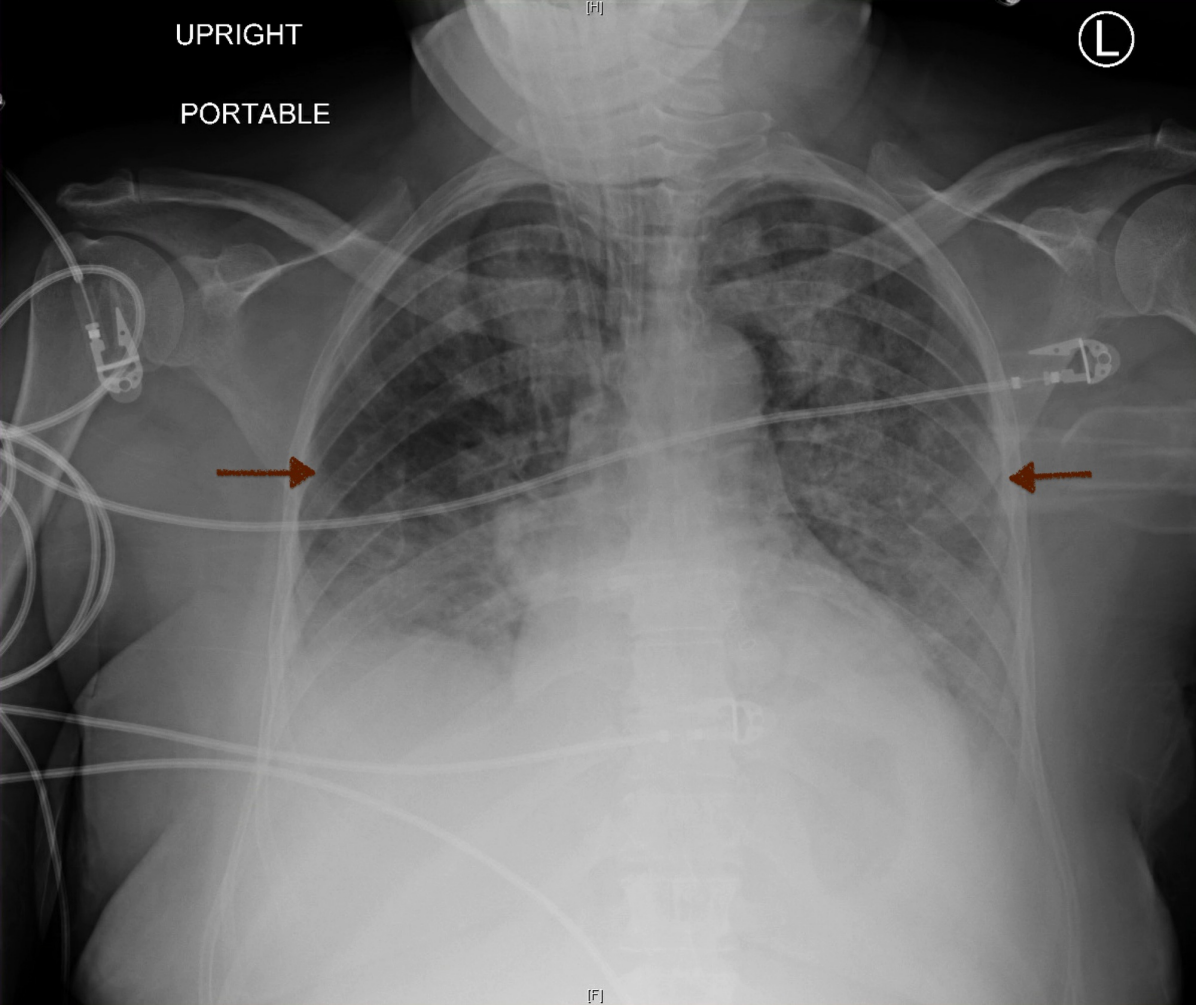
Chest X-Ray Improvement in aeration with a decrease in bilateral airspace infiltrates (red arrows). L: Left. H: Head. F: Feet.
